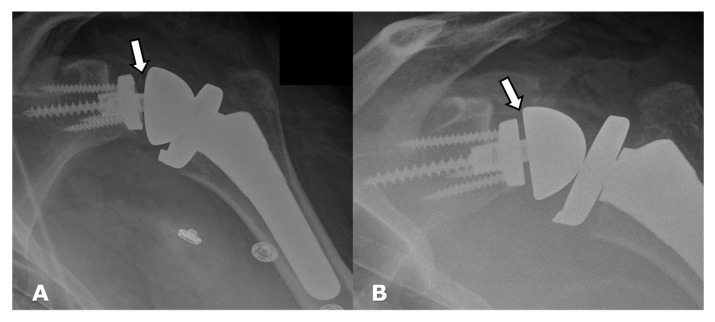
Frontal radiographs. (A). Immediate post-operative image following RTSA shows incomplete seating of the Morse taper into the baseplate, with resulting asymmetric alignment of the glenosphere (arrow). (B). Patient returned to the operating room for revision of the hardware, with post-operative image showing improved positioning of the Morse taper into the baseplate and improved glenosphere alignment.
